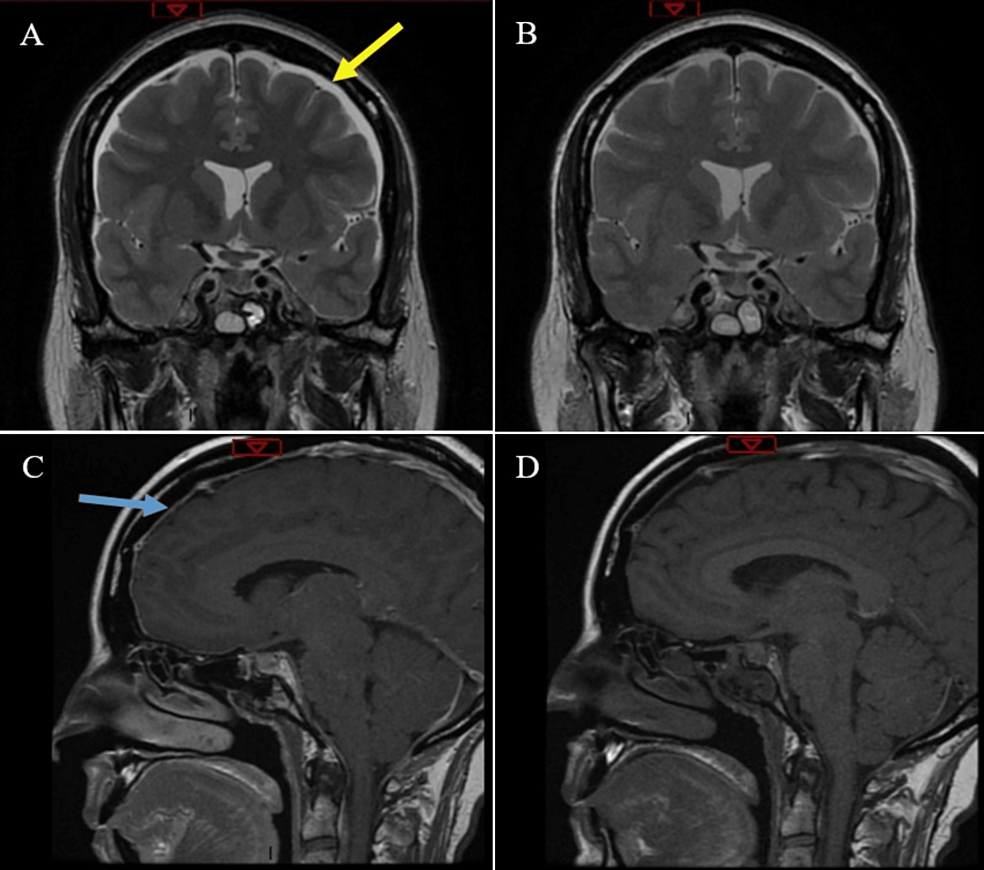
MRI brain demonstrating the presence and resolution of signs of intracranial hypotension Initial MRI demonstrating bilateral convexity subdural effusions (yellow arrow) with pachymeningeal enhancement (blue arrow) in coronal, T2-weighted (A) and sagittal, T1-weighted (C) images. Follow-up MRI three months later demonstrating resolution of above findings after the treatment of DI in coronal, T2-weighted (B) and sagittal, T1-weighted (D) images.
